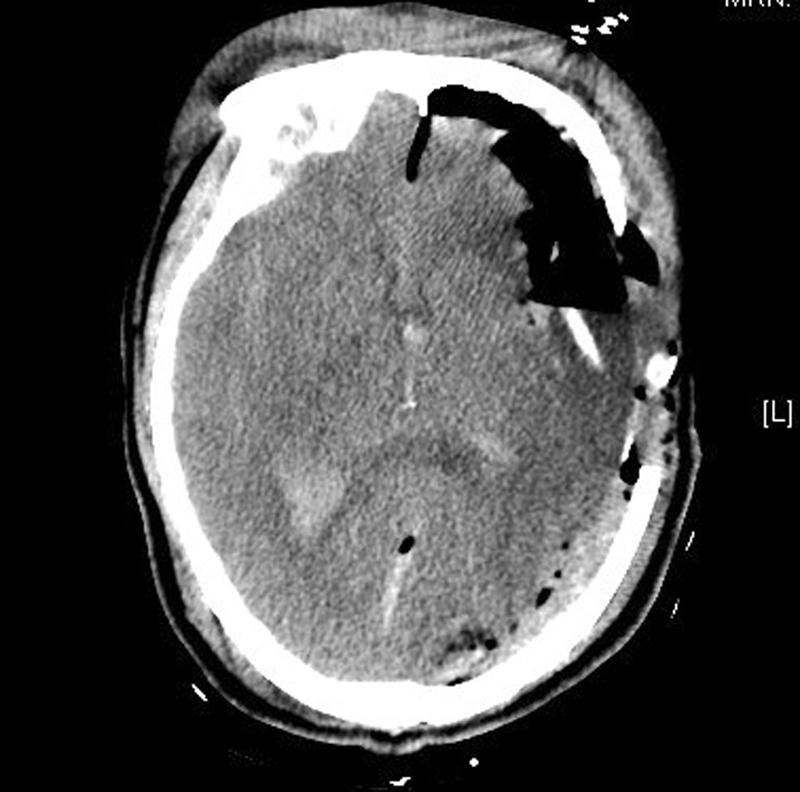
Computed tomography scan on the first day postoperation.
